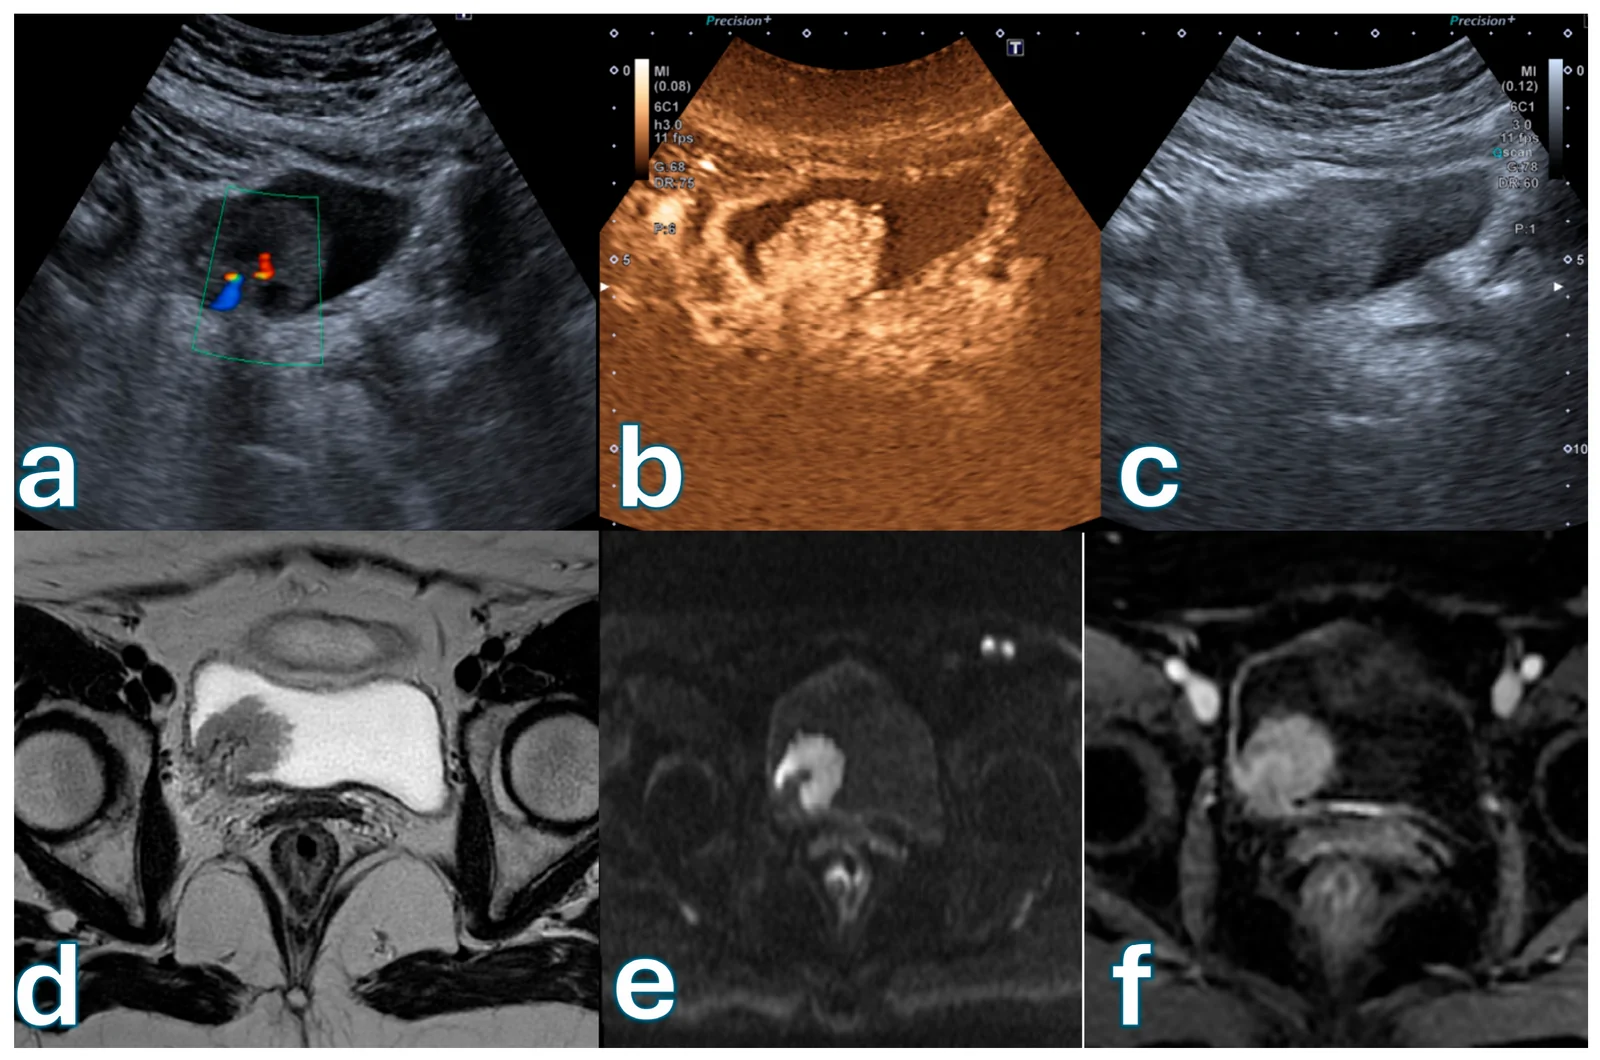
Multimodal imaging of a papillary bladder tumor demonstrating the added value of real-time CEUS for vascular stalk identification. (a) Color Doppler ultrasonography shows vascular signals at the tumor base. (b) During CEUS, a thin enhancing pedicle is visualized before complete tumor enhancement, with subsequent propagation of contrast from the pedicle into the lesion, fulfilling the definition of the CEUS vascular stalk sign. (c) Gray-scale ultrasonography shows the intravesical papillary mass. Corresponding multiparametric MRI images include axial T2-weighted imaging (d), diffusion-weighted imaging (e), and dynamic contrast-enhanced imaging (f). MRI depicts the tumor and its relationship with the bladder wall, whereas the vascular nature of the pedicle is more directly demonstrated by real-time CEUS.
